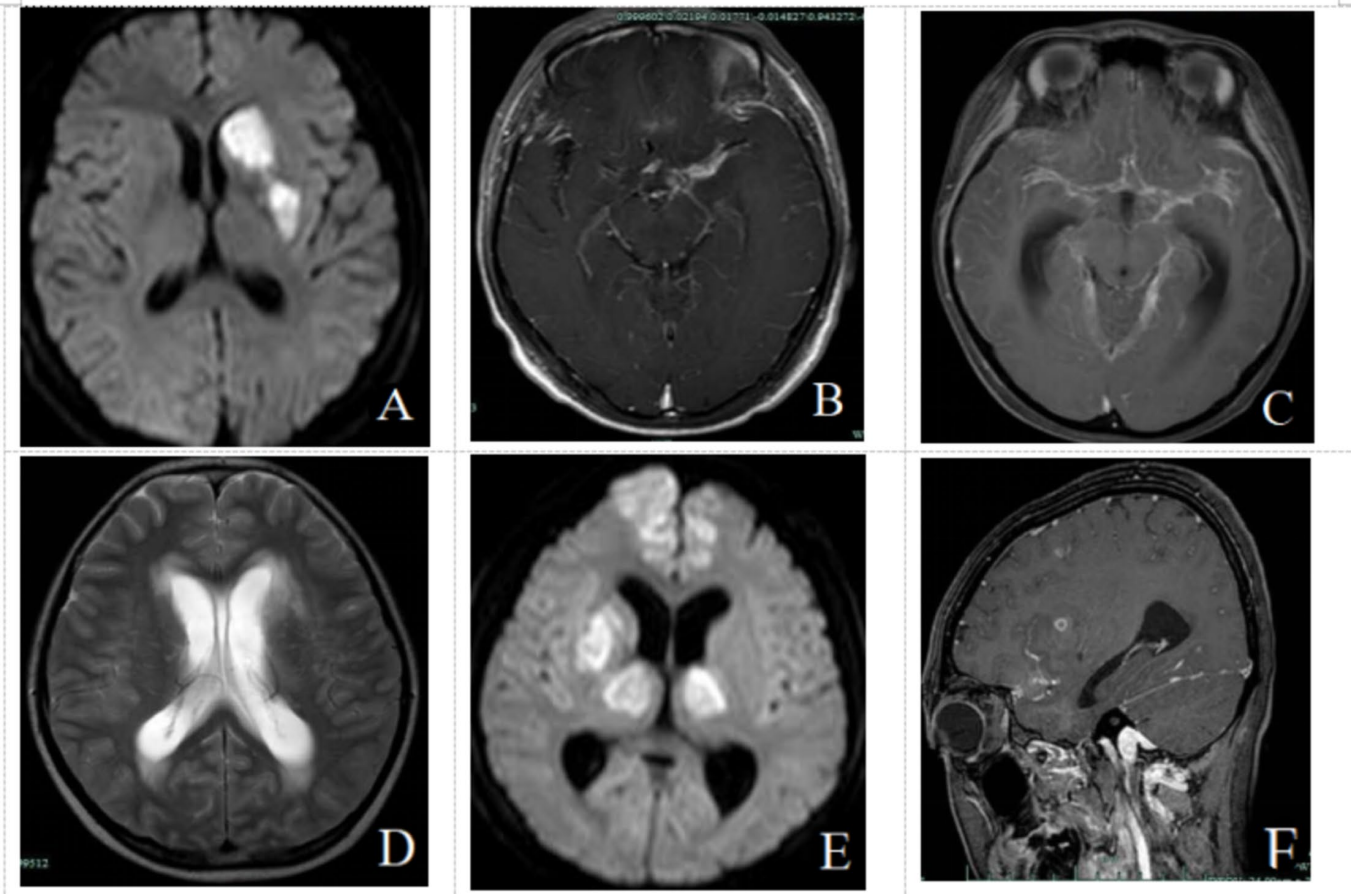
(A,B) MRI in a 23-year-old male patient with meningeal type intracranial TB. (A) DWI sequence shows acute infarction in the left basal ganglia. (B) Enhanced MRI shows M1 segment arteritis of left middle cerebral artery. (C,D) MRI in a 44-year-old man with mixed type intracranial TB. (C) Enhanced MRI showing changes in Willis ring arteritis. (D) T2WI shows Hydrocephalus. (E,F) MRI in a 23-year-old woman pregnant with mixed type intracranial TB. (E) DWI sequence shows multiple acute infarction in bilateral frontal lobes, right basal ganglia, and bilateral thalamus. (F) Sagittal enhanced MRI showing multiple lesions in the brain parenchyma and meninges.
